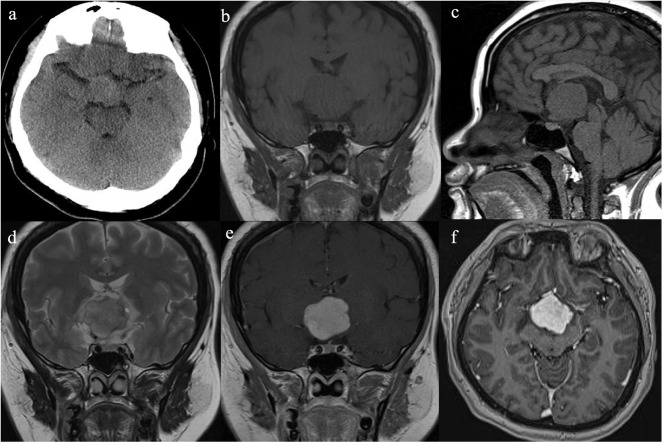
Radiological evaluation of CG preoperatively. A none-contrast CT scan showed a slightly hyper-dense mass located at suprasellar and the third ventricle (a). The tumor was slightly hypo-intense on Coronal and Sagittal T1WI (b,c) and slightly hyper-intense on Coronal T2WI (d). Post-contrast (gadolinium-enhanced) Coronal and Axial MRI (e,f) show prominent homogeneous enhancement.
